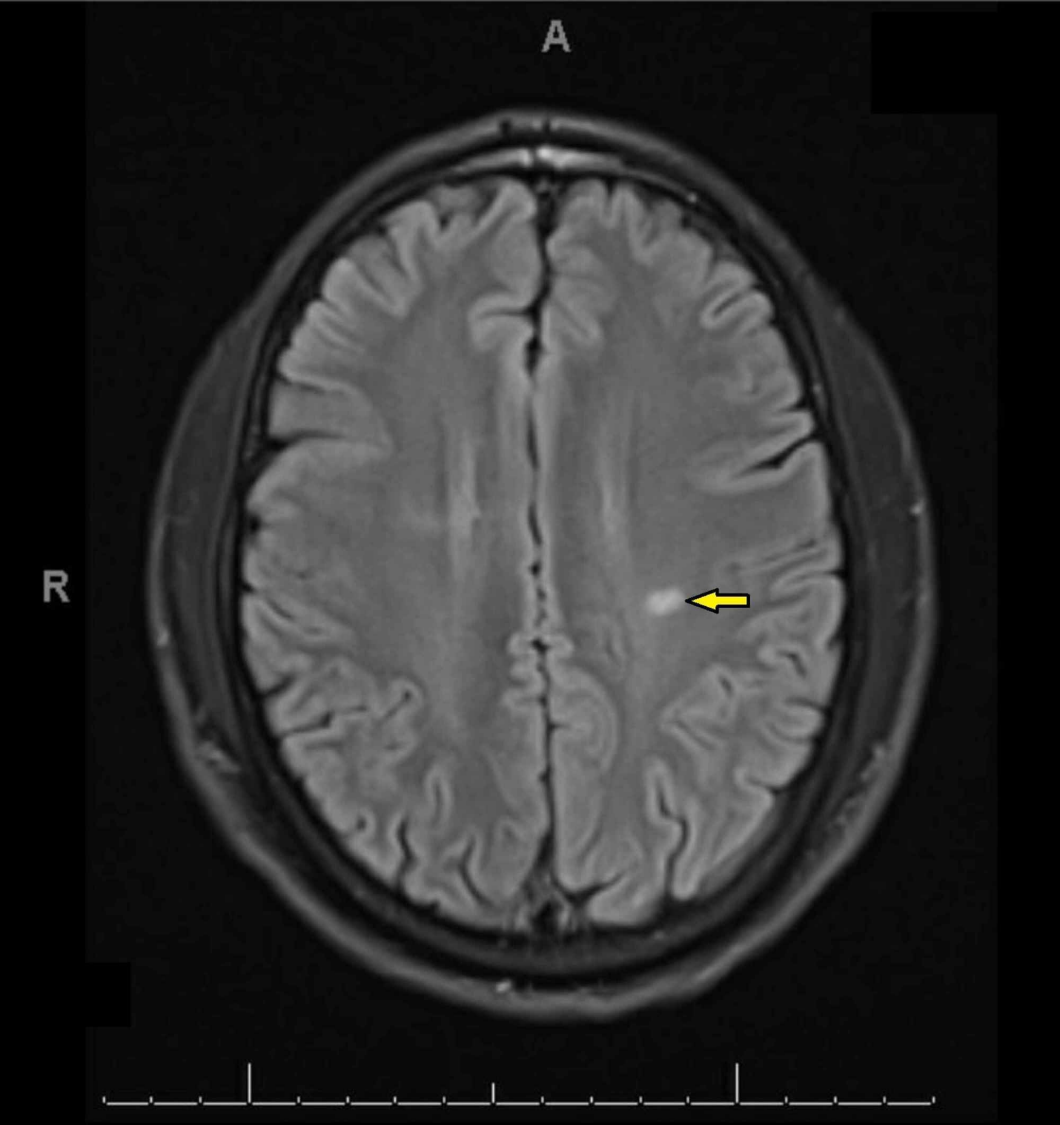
FLAIR/T2 weighted hyperintense signal foci in the left periventricular white matter on MRI.
